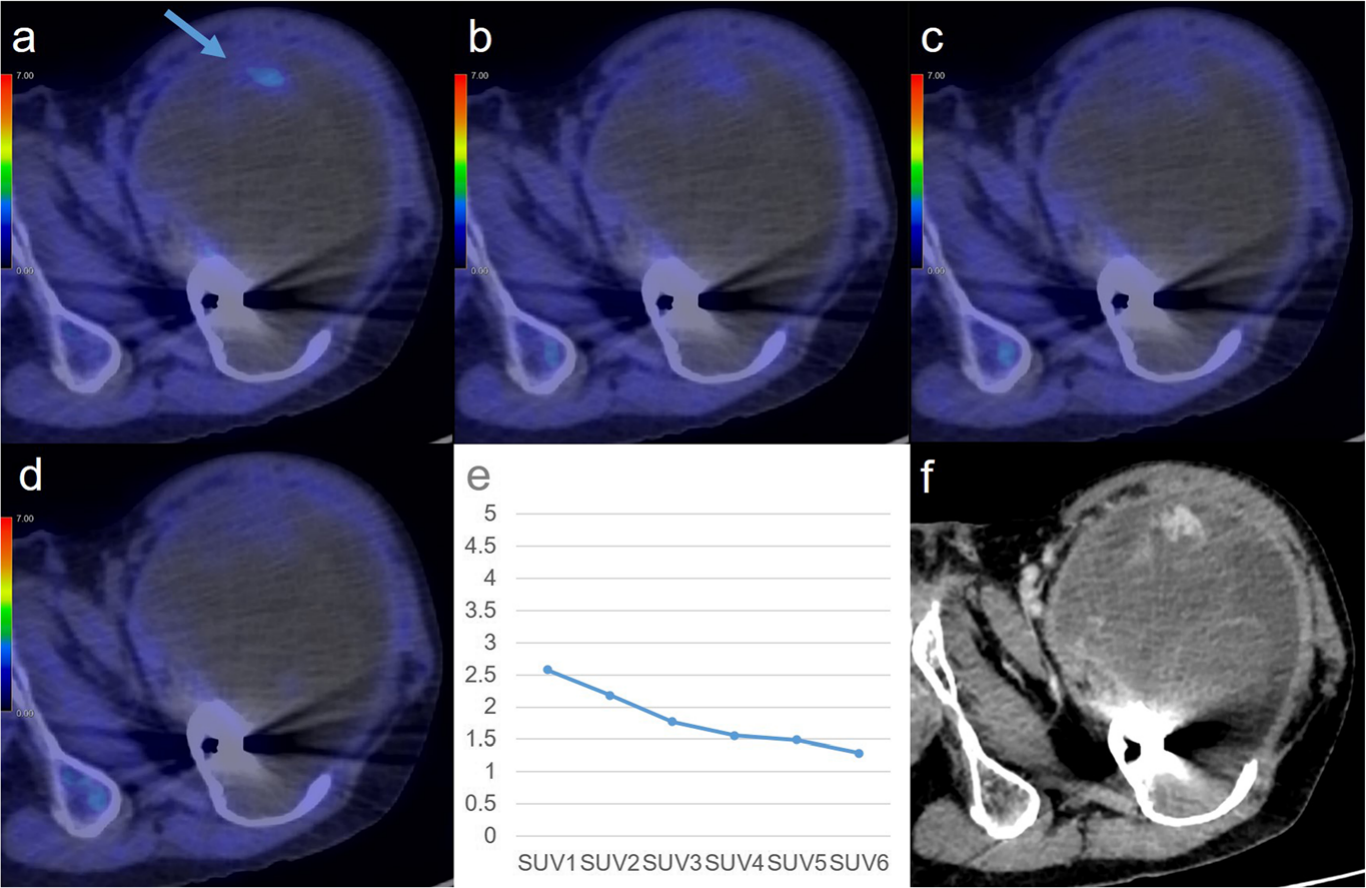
A 67-year-old man with chronic expanding haematoma in the right femur. Dynamic positron-emission tomography/computed tomography (PET/CT) (a–d) images are shown. In the dynamic first phases (a), the focal faint accumulation is identifiable in the anterior part from the dynamic first phase (arrows, SUVmax: dynamic first phase, 2.58). After the dynamic second phases (b–d), the accumulation gradually becomes subtler in most parts of the tumour (SUVmax: dynamic second phase (b), 2.18; dynamic third phase, 1.77; dynamic fourth phase (c), 1.56; dynamic fifth phase, 1.49; dynamic sixth phase (d), 1.29). The time-SUVmax curve (e) shows a low level in SUVmax at the dynamic first phase and a gradual decrease during the dynamic phases. The post-contrast CT with iodine contrast medium (f) reveals the enhancing solid component in the anterior part of the haematoma and cortical erosion of thigh bone by the haematoma
